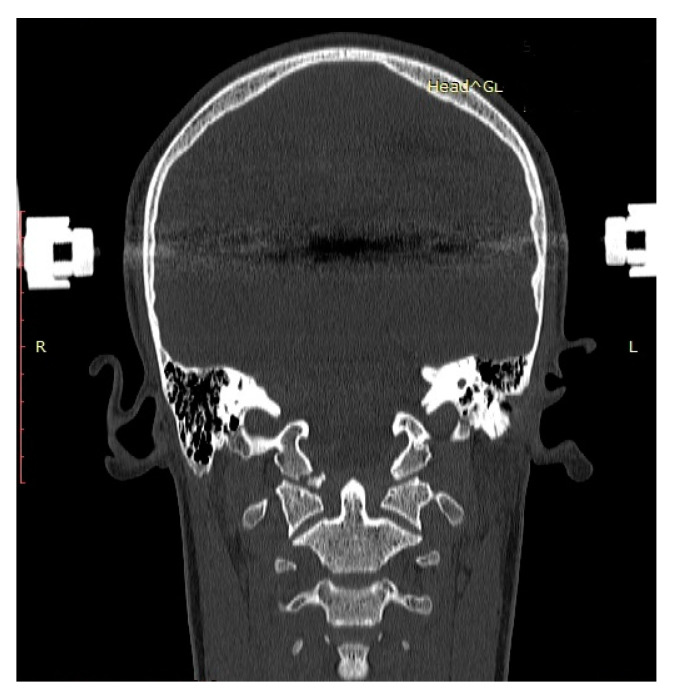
Control CT scan (frontal plane) of a boy aged 15.2 years (patient: P.P.) obtained after initiating halo-vest therapy and closed reduction of right-sided OCF. Correct alignment of condyle fragment on the right side and diminished fracture gap.
